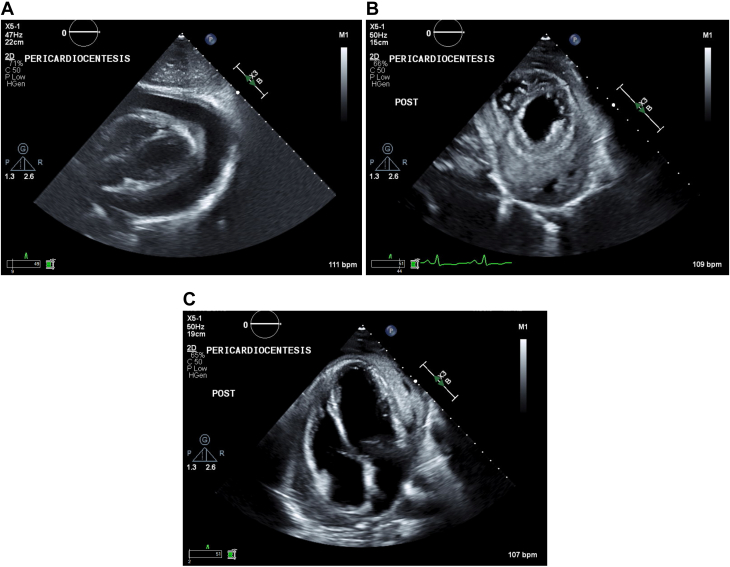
Periprocedural Echocardiography Transthoracic echocardiogram imaging obtained (A) immediately before pericardiocentesis and (B, C) immediately after pericardiocentesis and drain insertion, with visualization of remaining complex fluid collection.
